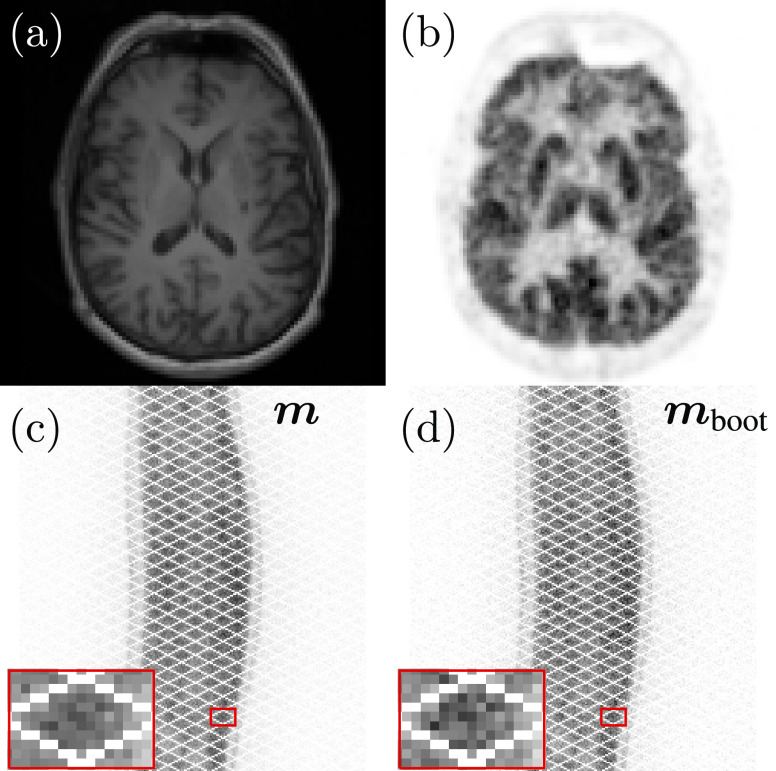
The patient PET-MR dataset used for the real data investigation. (a) The T1-weighted MR image resampled to the 3D PET image grid, (b) a slice of the 3D [^18^F]FDG image, reconstructed with 1000 iterations of MLEM followed by a 4mm FHWM Gaussian smooth, (c) a representative direct sinogram from the 3D [^18^F]FDG data acquisition (}{}$\boldsymbol {m}$), and (d) an example bootstrapped replicate of the same direct sinogram (}{}$\boldsymbol {m}_{\text {boot}}$). Red boxes in (c) and (d) show details of the sinograms for comparison.
